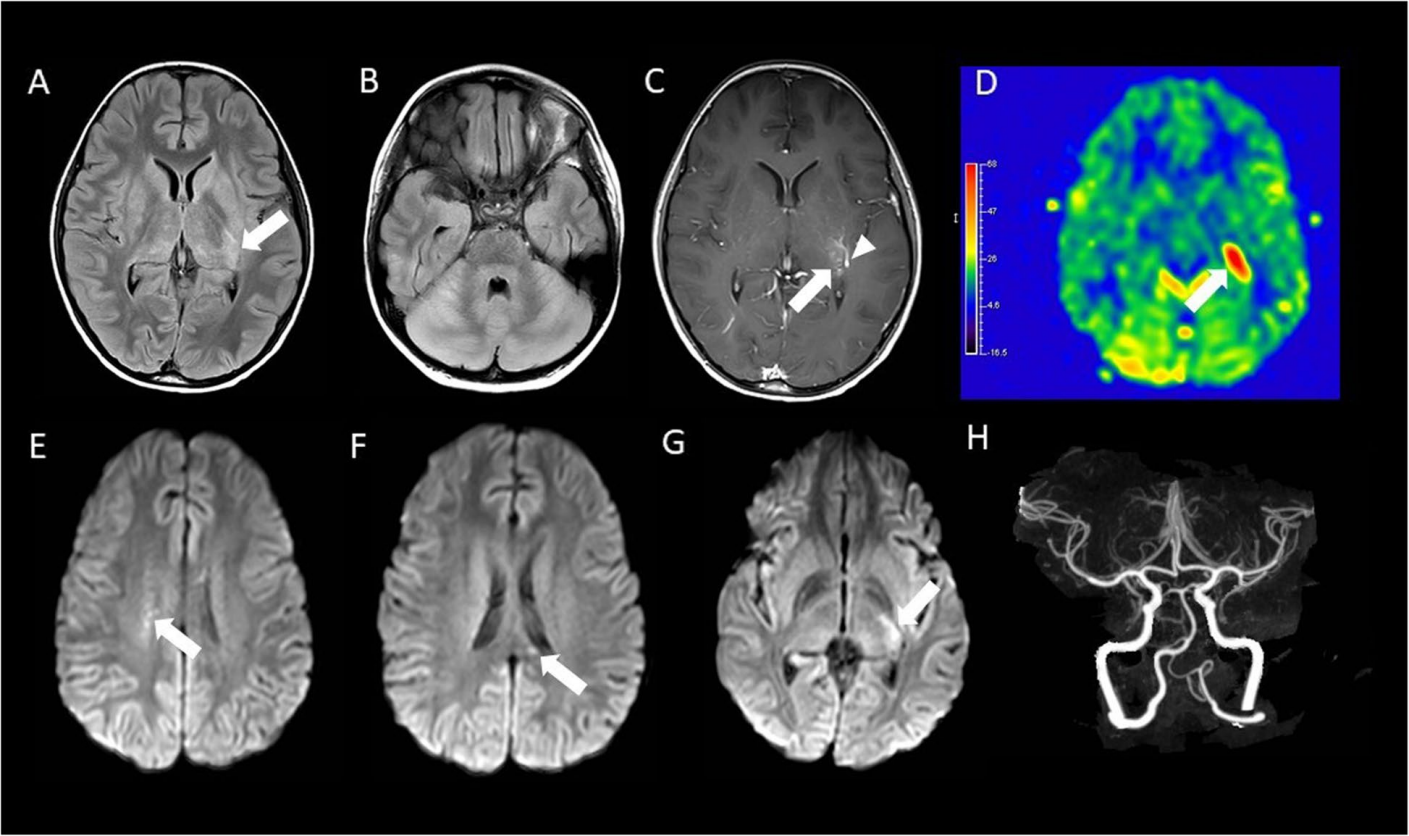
MRI of the brain obtained on day 11 of admission and CT angiogram of the head obtained on day 13 of admission. A and B. Axial pre-contrast FLAIR demonstrates increased conspicuity of the original focal thalamic lesion (arrow in A), as well as new, extensive abnormal FLAIR hyperintensity within the basal ganglia, thalami, cortex, brainstem, and cerebellum, with effacement of the cerebellar sulci C. Axial postcontrast T1-weighted imaging demonstrates increased enhancement of the lesion (arrow; noting incidental adjacent developmental venous anomaly, arrowhead). D. Axial arterial spin labeling image shows increased perfusion of the thalamic lesion. E–G. DWI images from superior (E) to inferior (G) demonstrate new lesions (arrows) in the right centrum semiovale (E) and splenium of corpus callosum (F), as well as increased size of the original focal thalamic lesion (G). H. Coronal maximum intensity projection image from a CT arteriogram of the head is normal
